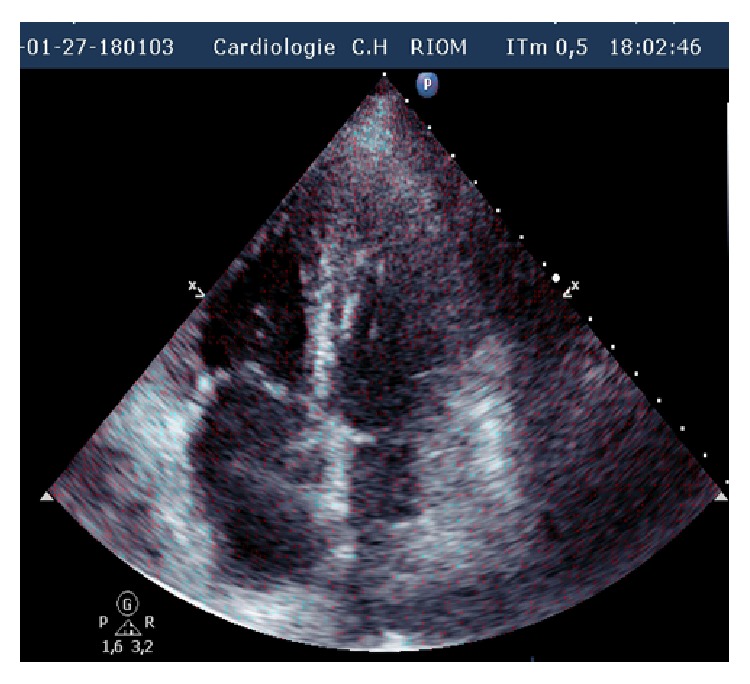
Apical four chamber view after thrombolysis showing complete disappearance of right heart thrombus.
